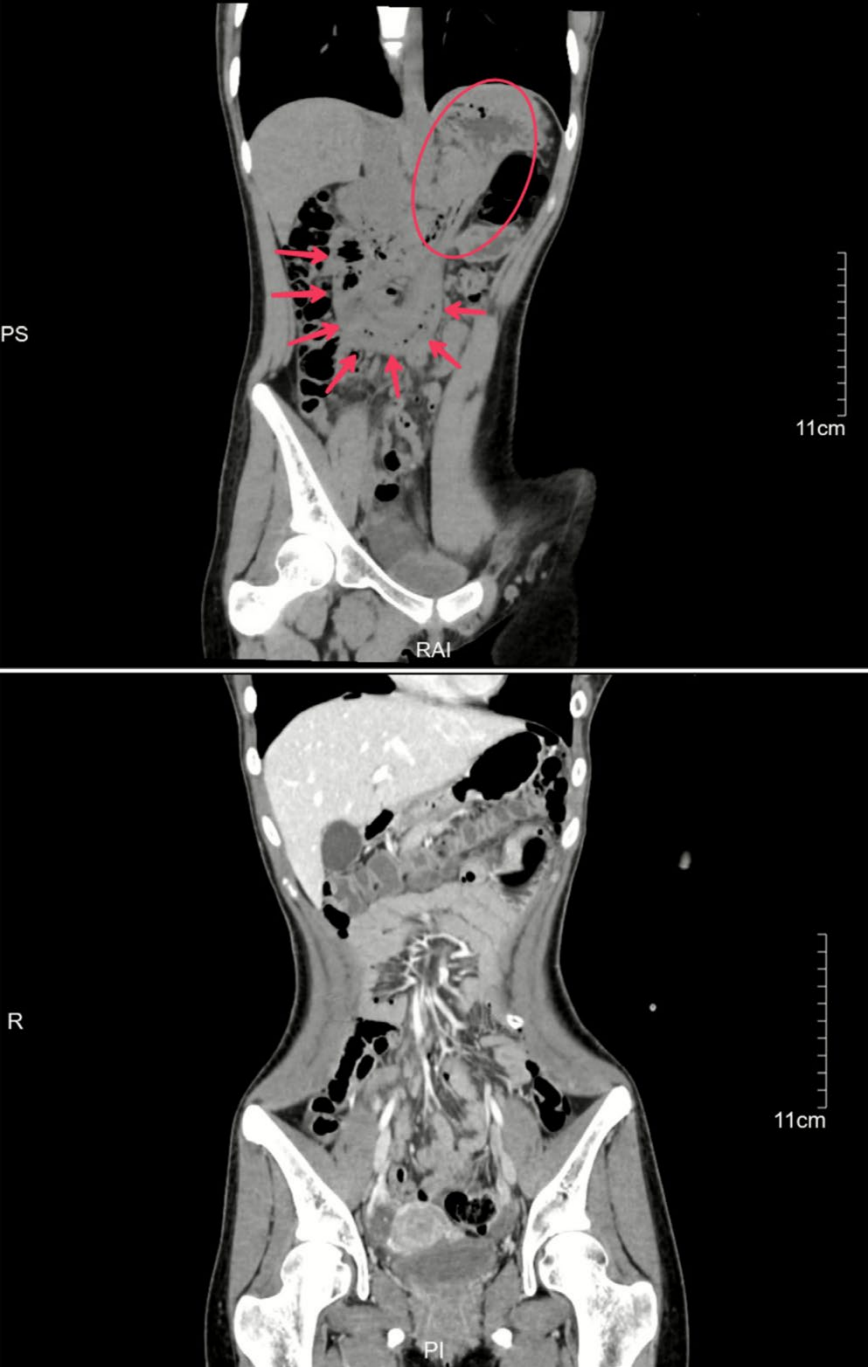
Comparison of coronal CT images before and after surgery: whirlpool‐like configuration of the small bowel (arrow) and associated traction on the gastric wall (circle).
